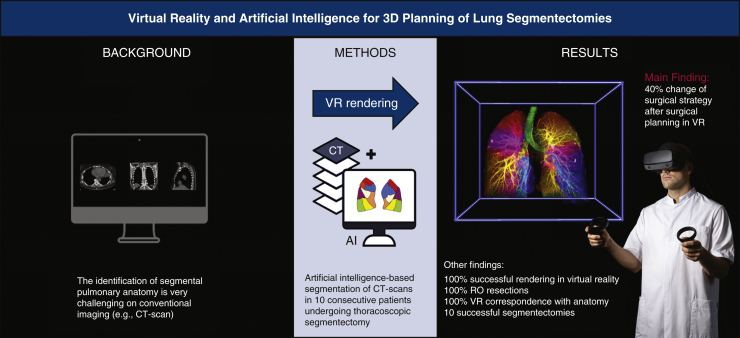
The identification of segmental anatomy for surgical planning of lung segmentectomy is quite challenging with conventional imaging. In the current study, a novel method is presented to create artificial intelligence based virtual reality reconstructions to prepare for thoracoscopic segmentectomies in 10 consecutive patients. *CT*, Computed tomography; *VR,* virtual reality.
